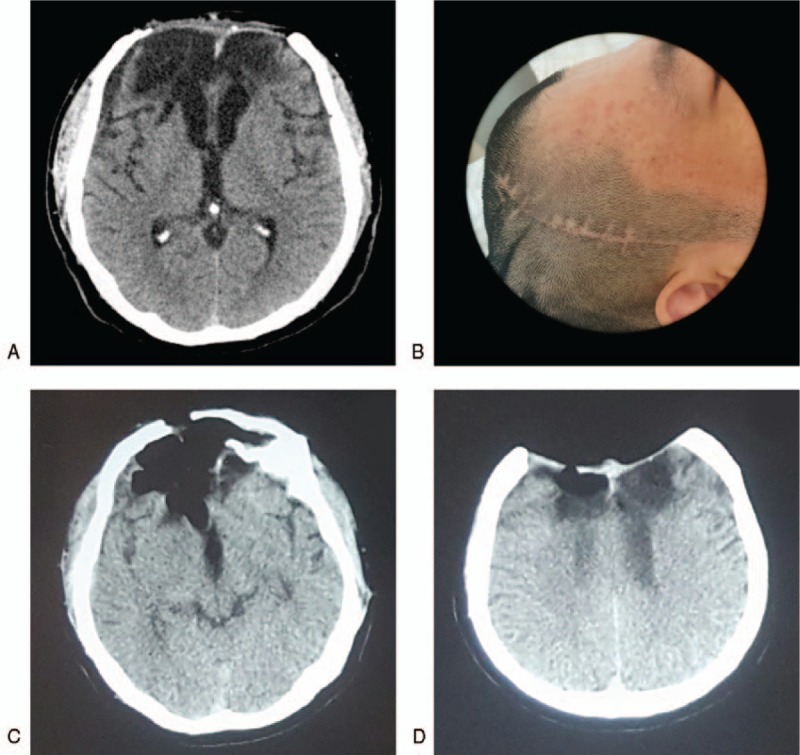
Images of the patient at admission. A: Head CT showed bilateral frontal bone defects and lobe necrosis; B: The surgical scar and a significantly sunken scalp were noted; C–D: Head CT showed intracranial pneumocephalus. CT = computerized tomography.
